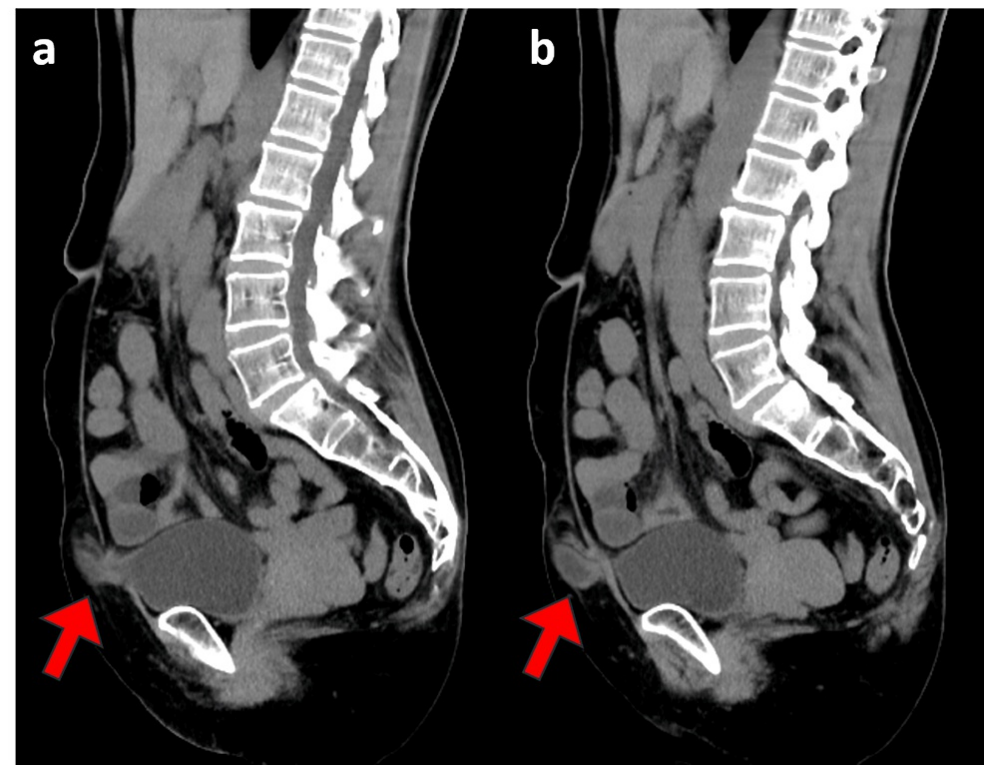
CT scan of the abdomen (sagittal). a, b: The abdominal incisional hernia in the left lower abdomen and a prolapsed bladder from the same area.
